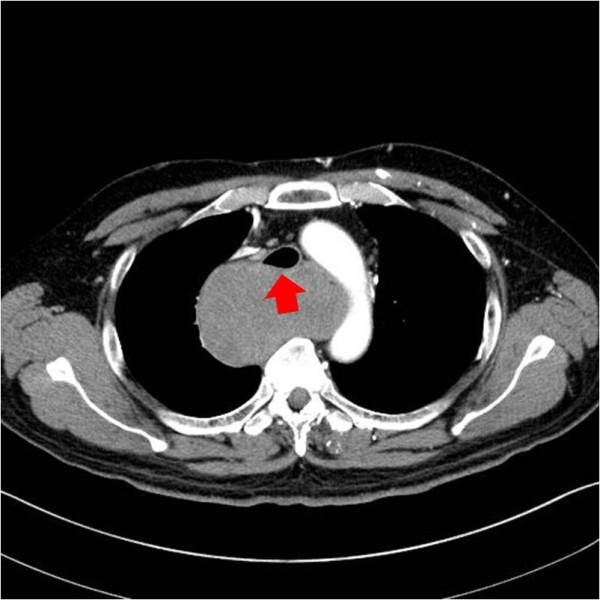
The chest CT revealed a heterogeneous large mass in the esophagus and tracheal compression (arrow).
